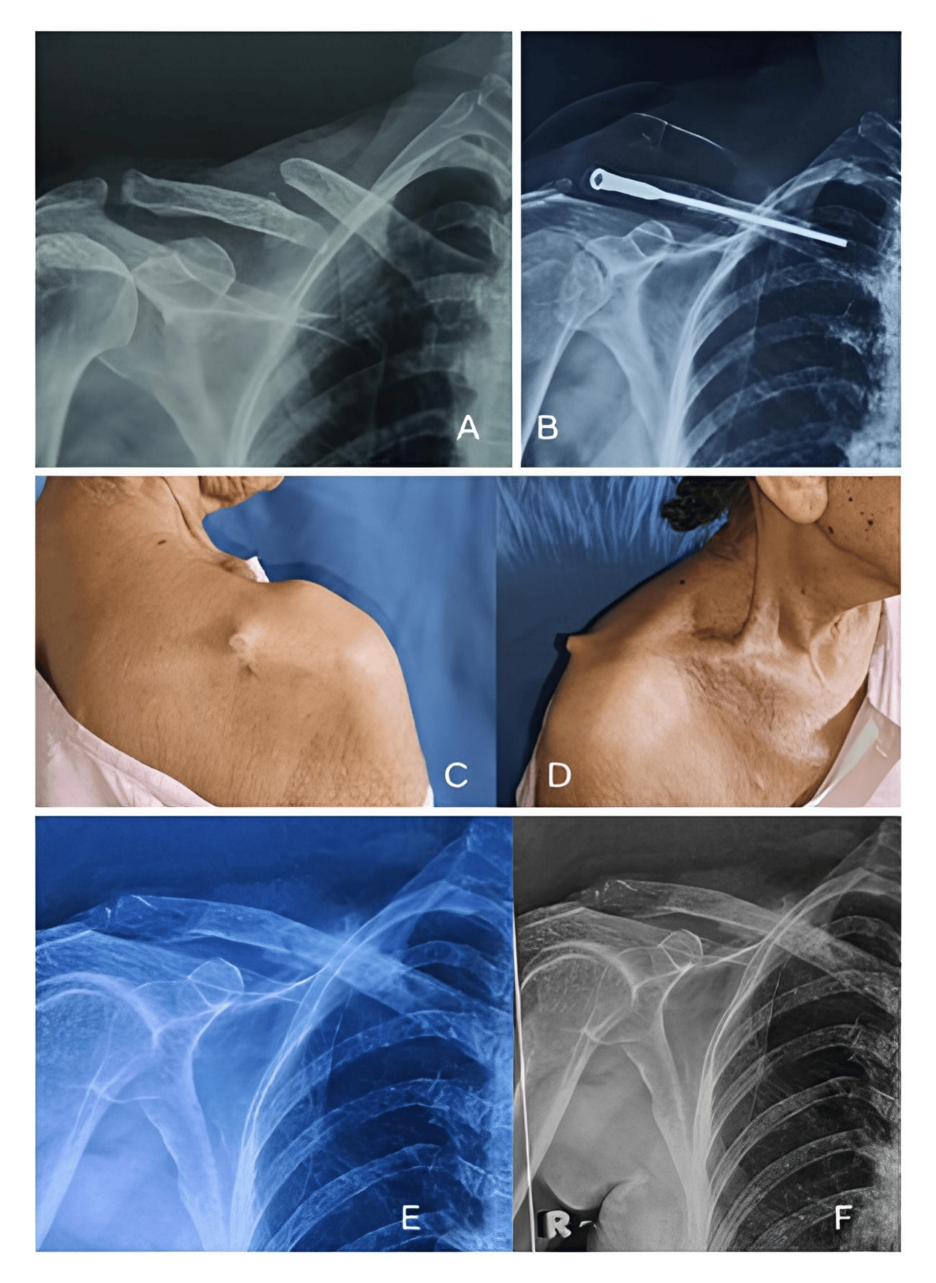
A 76-year-old female with a right middle-third displaced clavicle fracture treated with retrograde screw nailing. (A) Preoperative X-ray; (B) Postoperative X-ray; (C, D) Clinical images showing screw backout from the posterolateral aspect; (E) X-ray after removal of the screw nail; (F) X-ray 6 months after removal of the screw nail showing complete union of the clavicle fracture.
